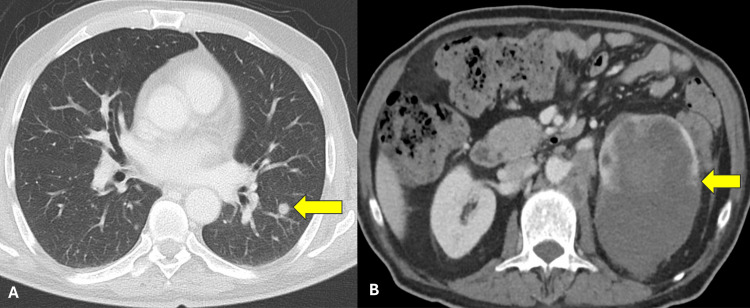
Computed tomography findings A: CT chest with intravenous contrast showing left pulmonary metastases (arrow); B: CT abdomen with intravenous contrast showing a Bosniak category IV left renal cystic mass invading the left adrenal gland (arrow) with multiple retroperitoneal lymphadenopathies
